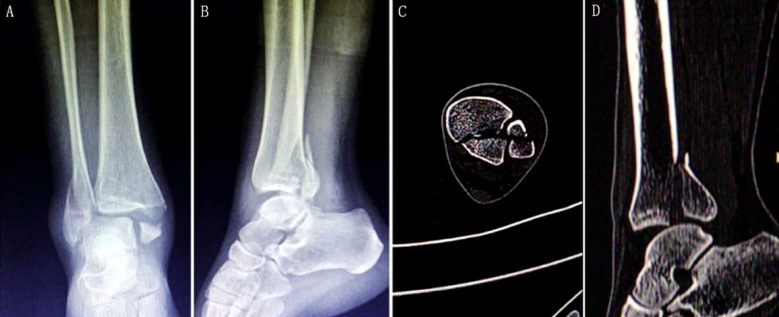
Imaging results a 47-year-old female patient who suffered from 3.5 h of car accident-induced right ankle swelling and pain complicated with limitation of motion before surgery. A: Frontal X-ray film of ankle joint; B: lateral X-ray film; C: CT scan cross-section image; D: CT scan sagittal image.
